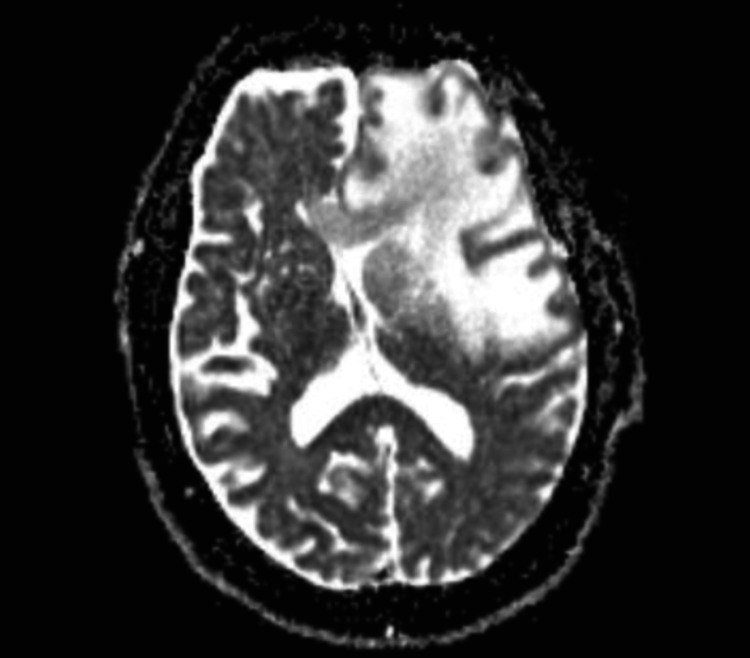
Brain MRI shows peripherally enhancing lesion noted anteriorly on the left extending to the cortical surface with new significant surrounding vasogenic edema
